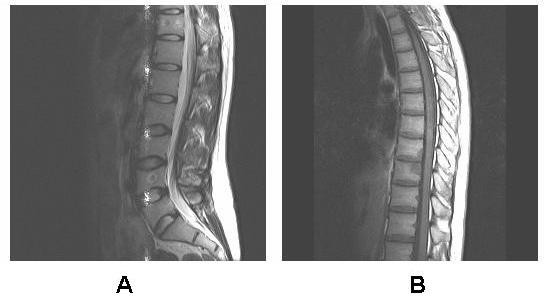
Magnetic resonance imaging shows metastatic osteoblastic lesions of the thoracic and lumbar vertebrae 3 months after operation.
